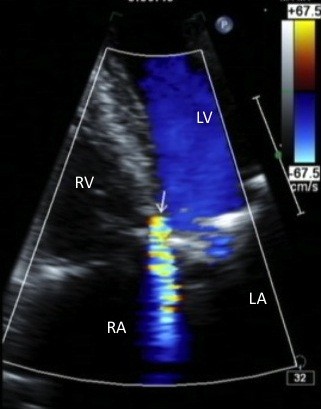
Preoperative echocardiography showing a high-velocity jet (arrow) originating from the left ventricular outflow tract and extending to the right atrium along the tricuspid valve leaflet. LA: left atrium; LV: left ventricle; RA: right atrium; RV: right ventricle; TV, tricuspid valve.
